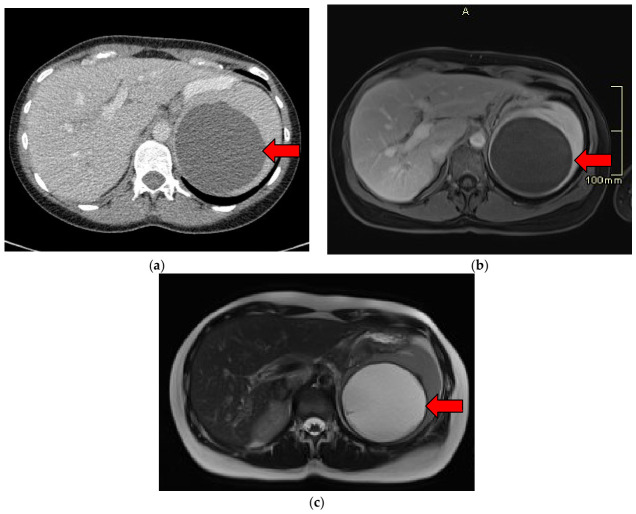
10 cm epithelial cyst in a 35-year-old female. (a) On this axial contrast-enhanced CT, the cyst is a homogenous thin-walled mass that is well circumscribed. (b) On this axial T1-weighted image, the cyst is a thin-walled, well-circumscribed mass that has homogeneously low signal intensity. (c) On this axial T2-weighted image, the cyst is a thin-walled, well-circumscribed mass with a homogeneously high signal.
